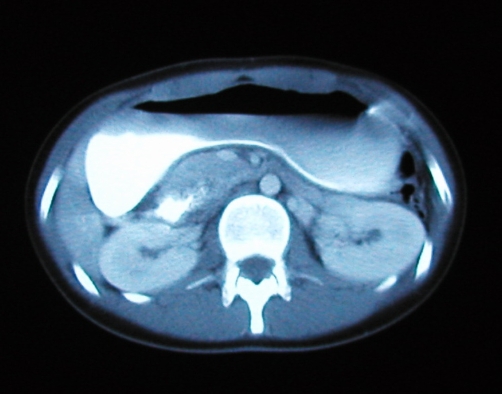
CT showing gastric dilatation.
